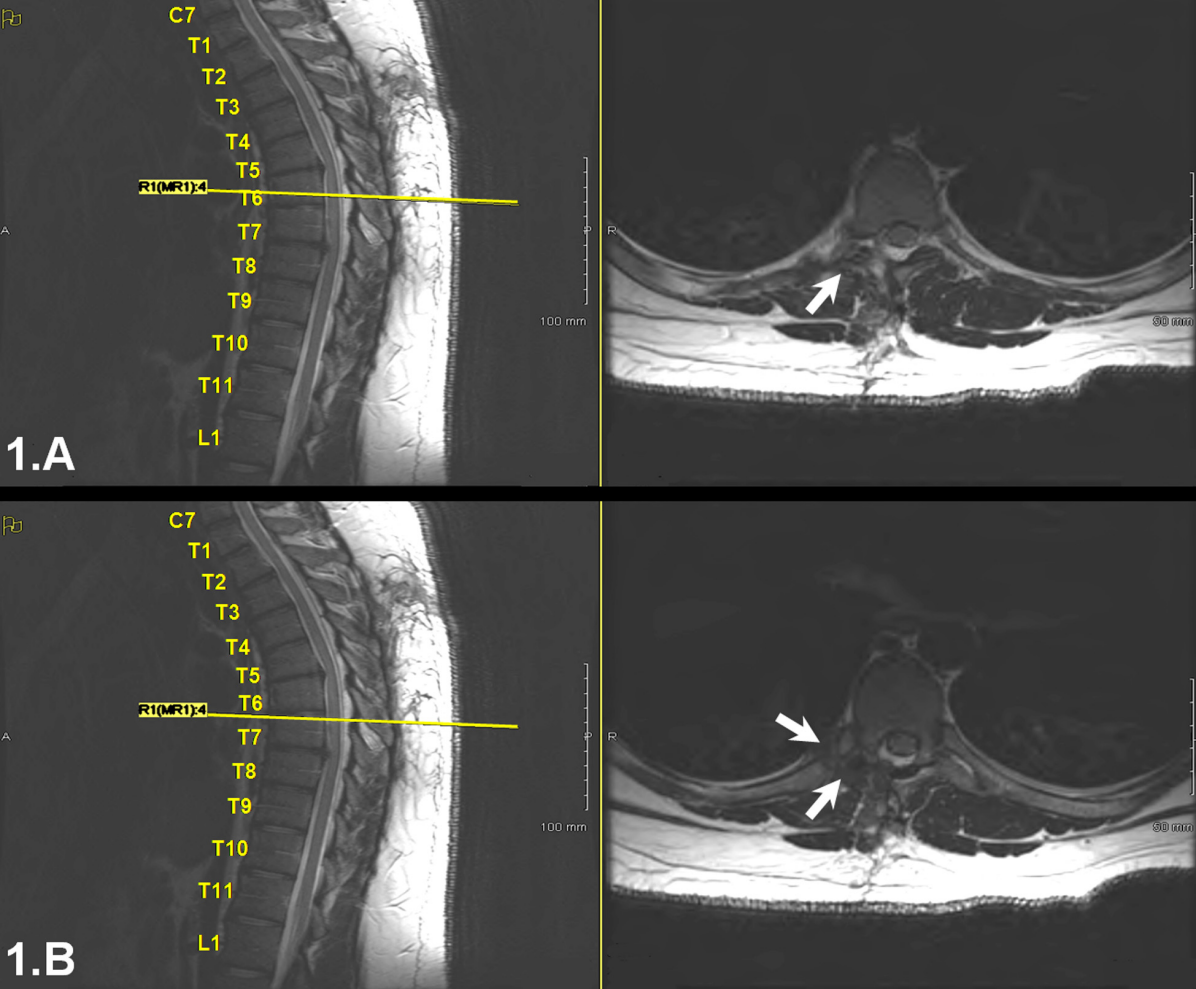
MRI imaging after the first discectomy and decompression. A. complete right sided laminectomy at T6-7 (arrow). B. partial laminectomy at T7, a fractured right T7 rib (double arrow).
